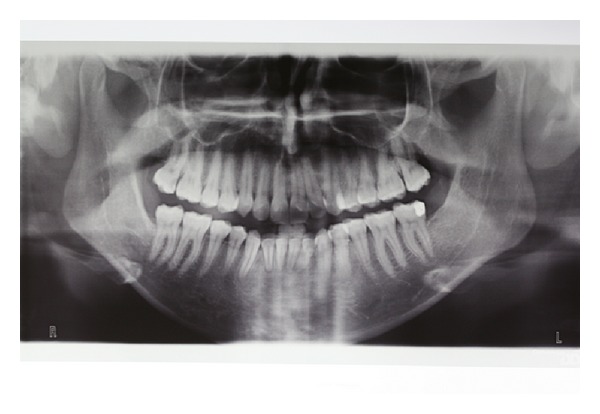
Orthopantomography at 1 year.
